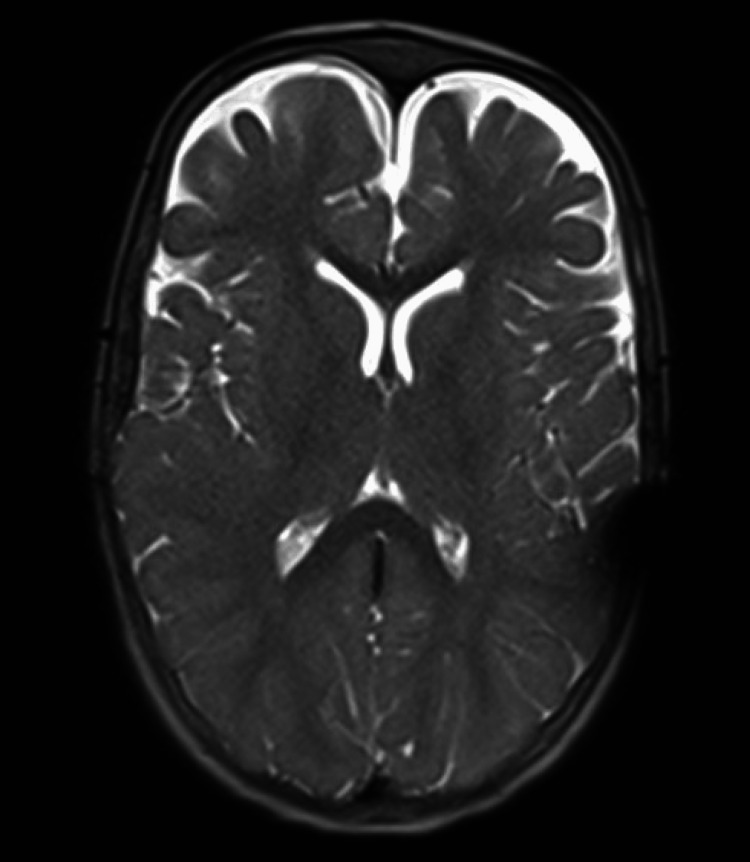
Postoperative MRI showing interval improvement of bilateral subdural hygroma after the insertion of a subdural-peritoneal shunt.
